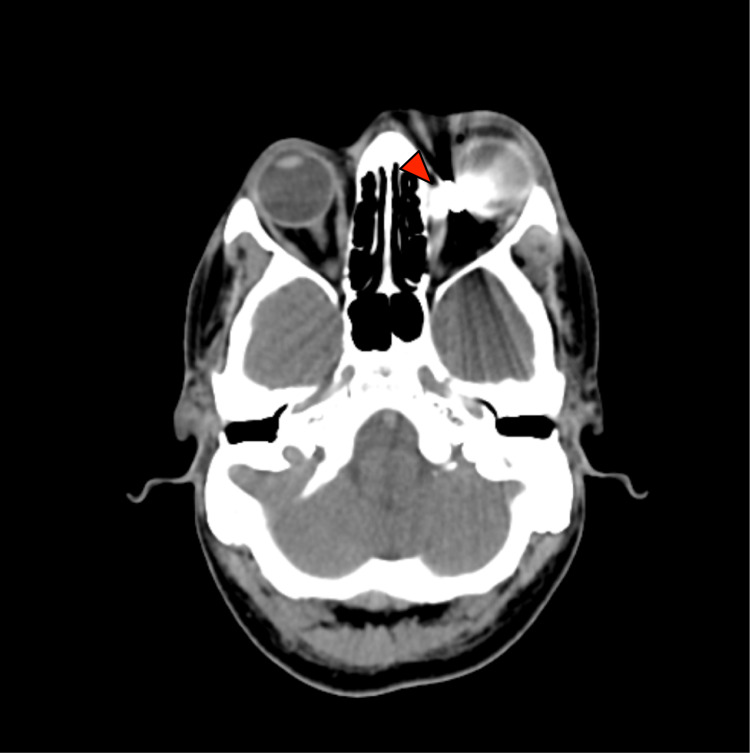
CT imaging demonstrating a foreign body (arrow)
